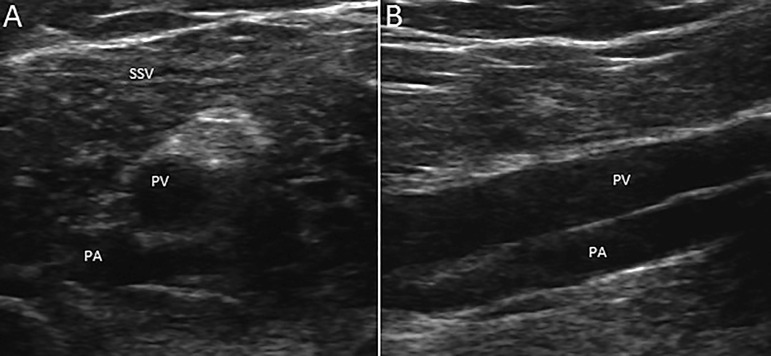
A and B. Venous duplex ultrasound B-mode images, axial and sagittal views. PA, Popliteal artery. PV, popliteal vein. SSV, small saphenous vein.
